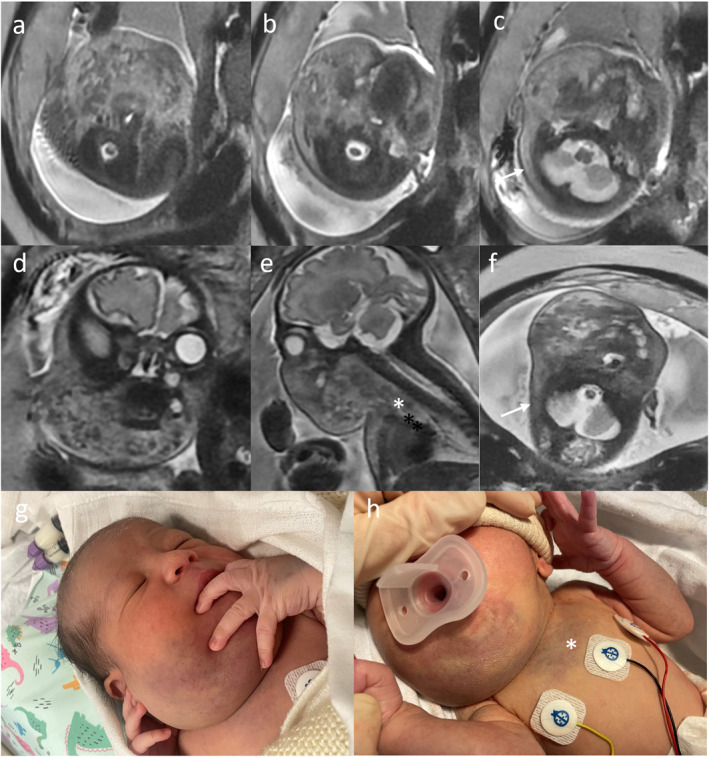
(a–h, Case 4). Axial and coronal T2‐weighted fetal MRI at 32 weeks' gestation (a, d) shows a large bilateral submandibular hyperintense infiltrative lesion involving both subcutaneous and deep soft tissues of the lower face (sublingual and right parotid regions), with right‐sided predominance and extension into the right posterior cervical tissues (c, arrow). Additional anterior thoracic wall involvement is visible on sagittal MRI (e, *). Follow‐up axial MRI 3 weeks after initiation of maternal sirolimus therapy demonstrates decreased extent of infiltration and reduced T2‐signal intensity in the right cheek and right posterior cervical tissues (f). Clinical examination at birth confirmed right lower‐face (g) and anterior thoracic wall infiltration (h), with violaceous skin discoloration that was less marked than in Figure 2f at the onset of KMP. Laboratory tests showed thrombocytopenia (30 G/L), consistent with ongoing KMP.
